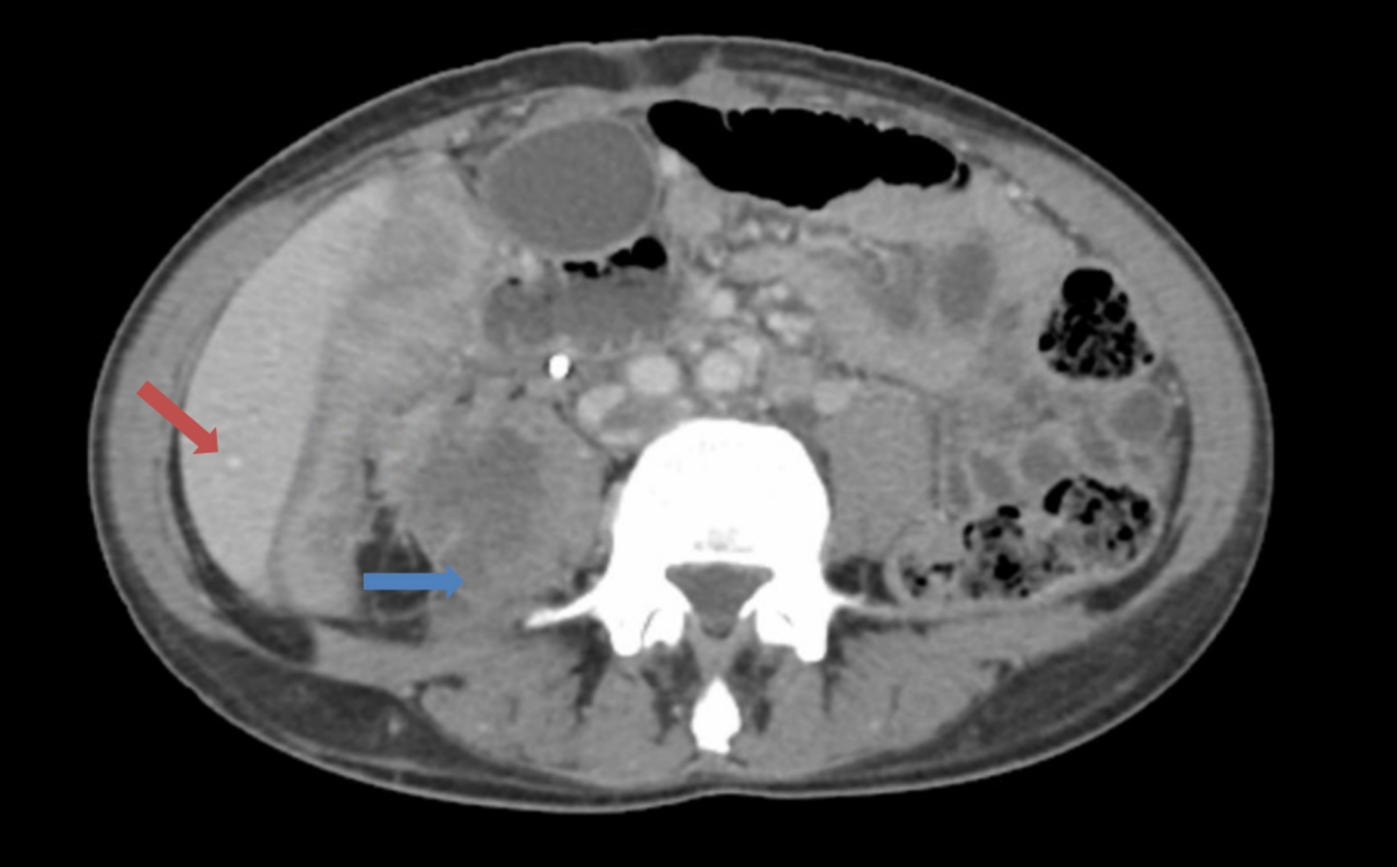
Computed tomography (CT) of abdomen showing multiple metastasis. CT image showing 1) multiple new hepatic lesions, likely metastases (red arrow) and 2) heterogeneous lesion involving the lower pole of the right kidney with extension into the right psoas muscle (blue arrow).
